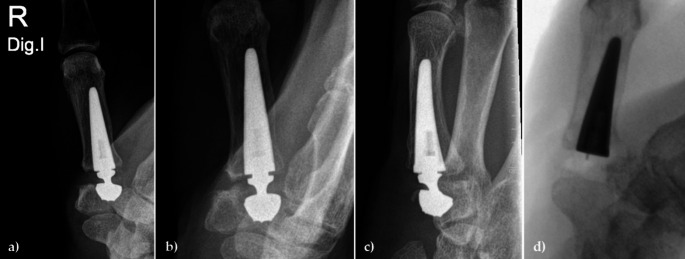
Radiographs of a patient before and after revision surgery. a) Radiograph after initial surgery showing primary cup misplacement; b) and c) Cup dislocation 2 years after initial surgery; d) Revision surgery performed as secondary trapeziectomy
